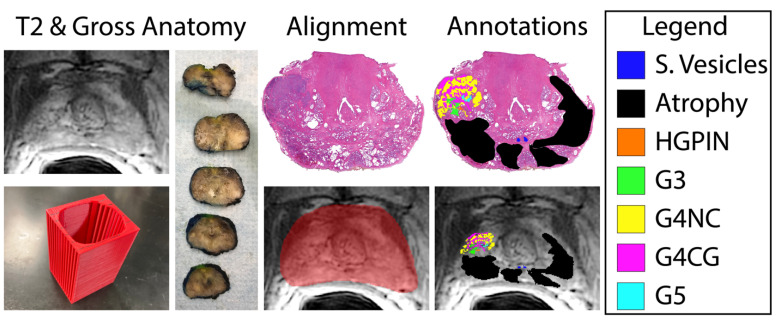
Tissue processing and MRI co-registration process. Slicing jigs (bottom, left) are 3D modeled to section tissue in line with the slice thickness and orientation of each patient’s T2-weighted image (left). Slides are digitized and annotated by Gleason pattern (legend, (right)) and aligned to the corresponding slice on the T2WI (bottom, middle).
